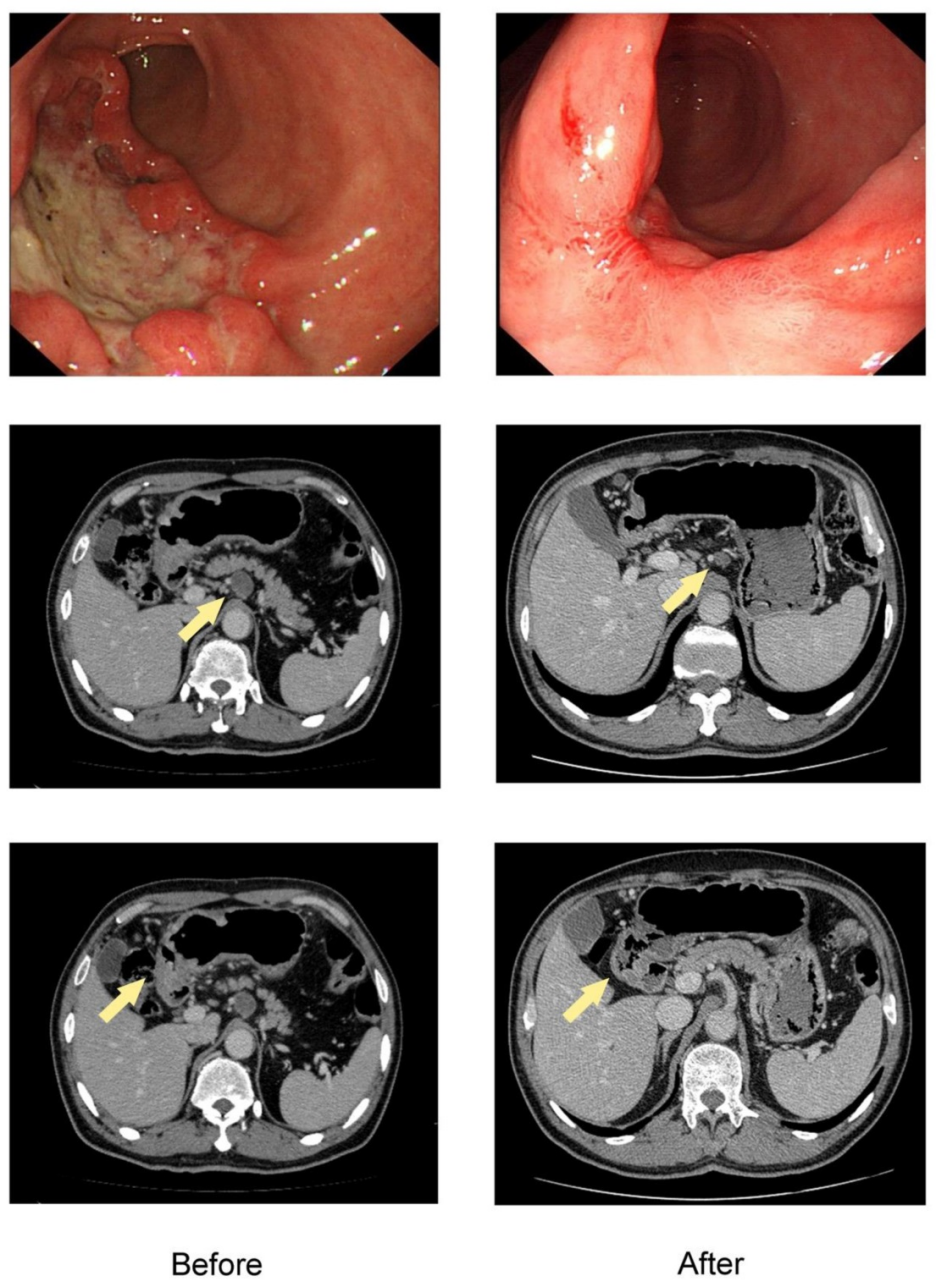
The endoscopic and CT images of the representative patient treated with conversion therapy. Baseline (left) and after 4 cycles of SOX chemotherapy (right) of the primary tumor and ERLN. The lesion of this patient was initially defined as unresectable due to ERLN+. The shrinkage was obtained both in the primary tumor and ERLN and the response was considered to be a PR, which was confirmed by the RECIST criteria.
